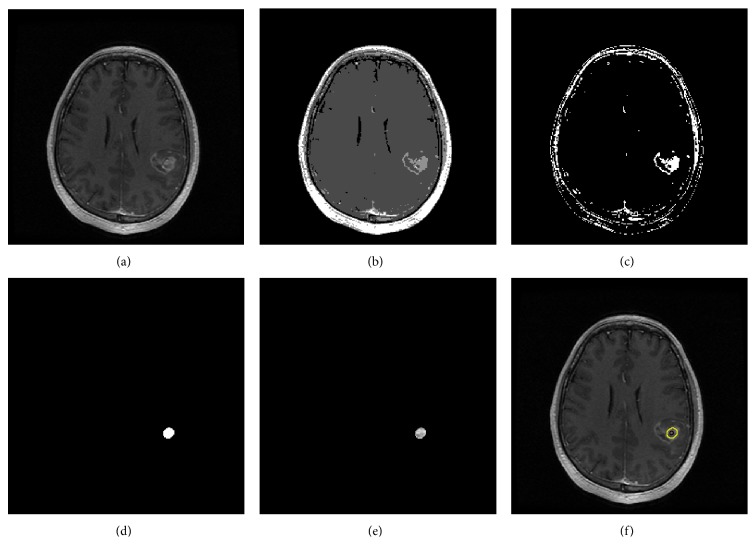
GBM detection by segmentation and morphology operations: (a) T1-MR image, (b) image segmented by four levels, (c) range of GBM gray level conserve, (d) filtering of (c), (e) raw GBM data detected, and (f) GBM located on the brain image.
